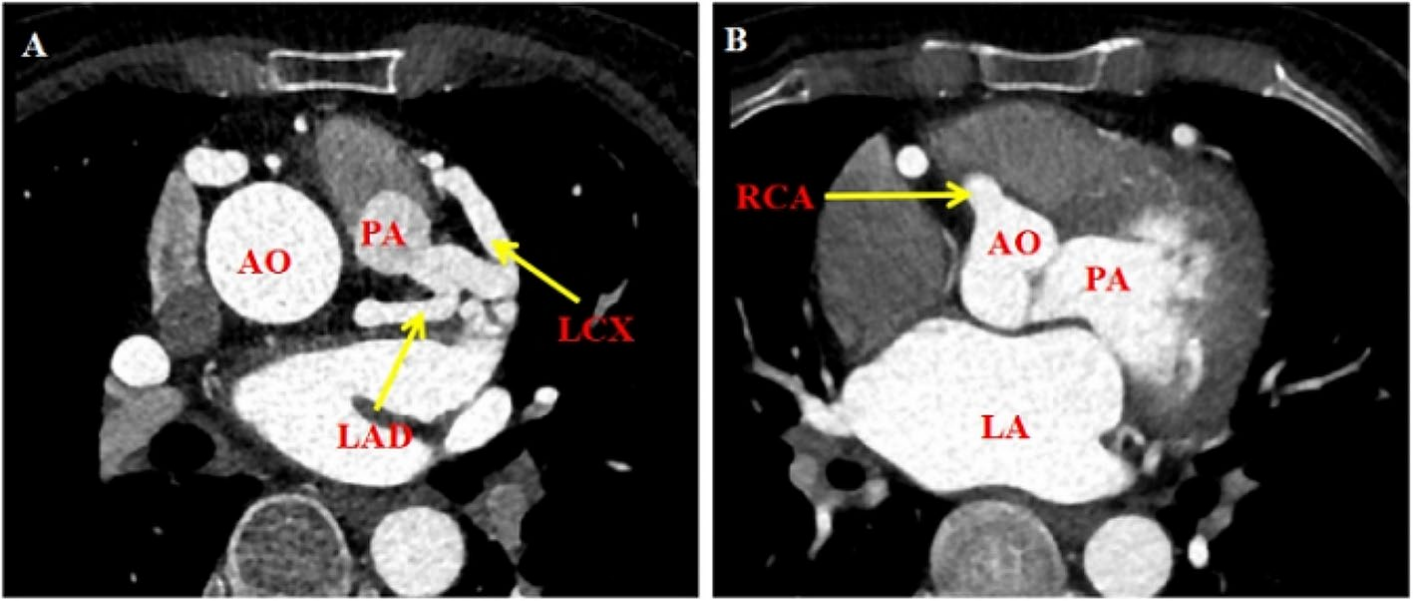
Preoperative coronary computed tomographic angiography. A The ectopic left coronary artery originated from the pulmonary artery. B The right coronary artery originates from the right aortic sinus. AO: aorta, PA: pulmonary artery, LA: left atrium, LAD: left anterior descending branch, LCX: left circumflex branch, RCA: right coronary artery
